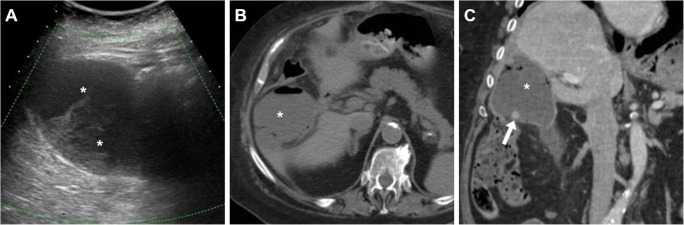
Gallbladder fossa abscess (*) observed after open cholecystectomy converted from laparoscopic cholecystectomy because of gallbladder perforation and intraperitoneal spillage of infected bile, sonographically (a) seen as ovoid well-demarcated infrahepatic collection with inhomogeneous hypo-anechoic structure. Unenhanced (b) and post-contrast (c) CT images confirmed abscess collection occupying the surgical bed, with predominantly fluid content, non-dependent air and thin enhancing peripheral wall. The faintly calcific fragment (*arrow* in c) corresponded to a dropped gallstone. Percutaneous CT-guided drainage was required to relieve extended-spectrum beta-lactamase-producing (ESBL+) *Escherichia coli* infection. (Partially reproduced from Open Access ref. [43])
